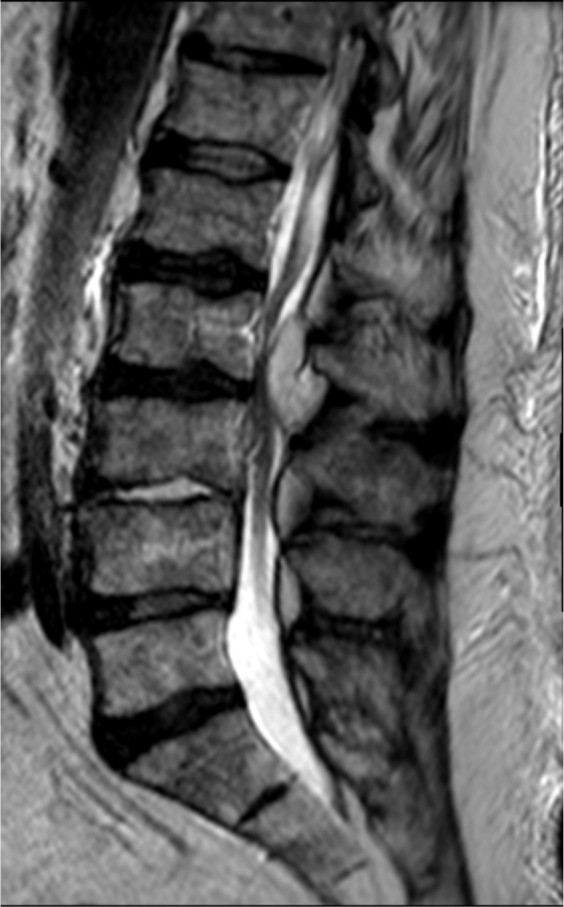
Sagittal T2 MR image of the lumbar spine again demonstrating
